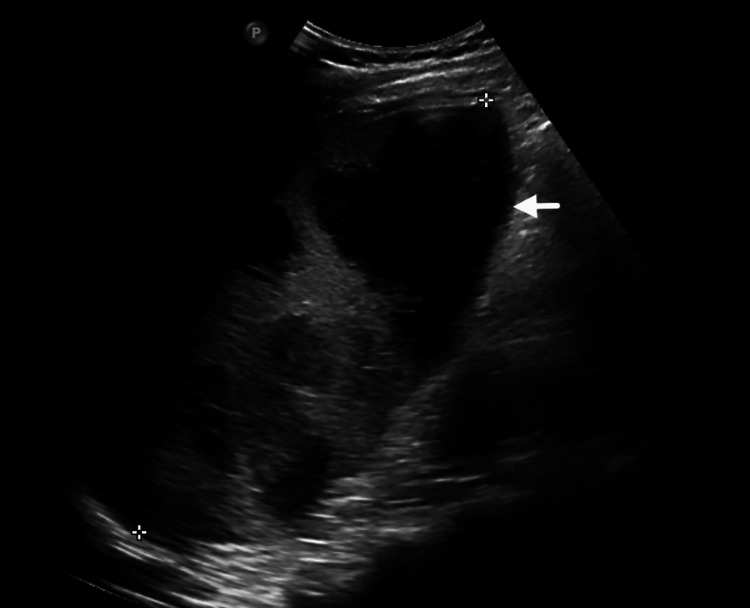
USG abdomen showing splenomegaly with a large, lobulated and partially liquefied abscess USG: ultrasonography.
